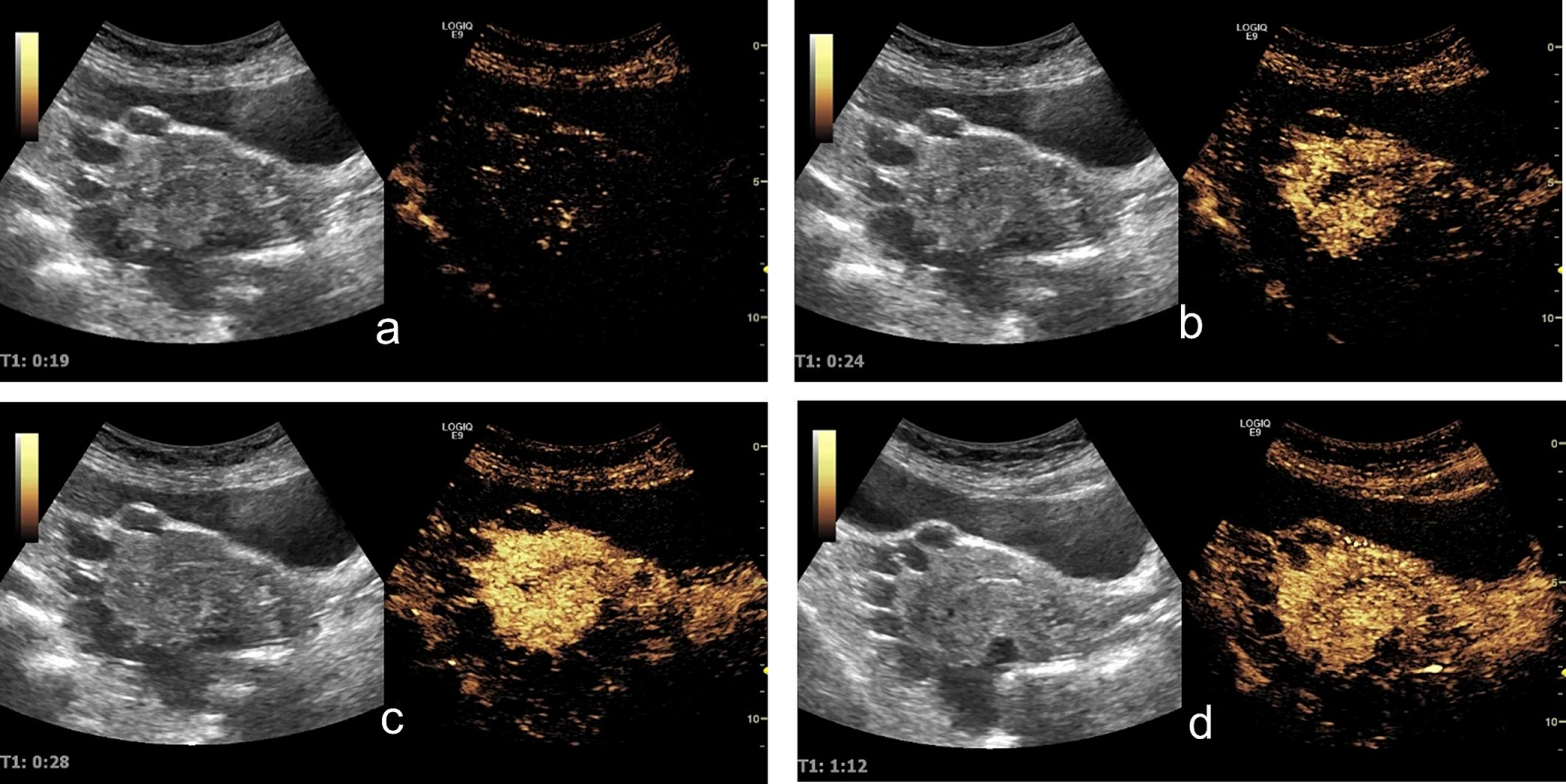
Contrast-enhanced ultrasound findings: (a) At 19 s, the uterine myometrium begins to enhance. (b) At 24 s, the uterine myometrium shows significant enhancement, while no obvious enhancement is observed in the lesion walls or the endometrium. (c) At 28 s, the lesion walls and endometrium begin to enhance, with the uterine myometrium showing marked enhancement. (d) At 72 s: The contrast agent demonstrated a washout phase in the lesion capsule wall, endometrium, and myometrium.
